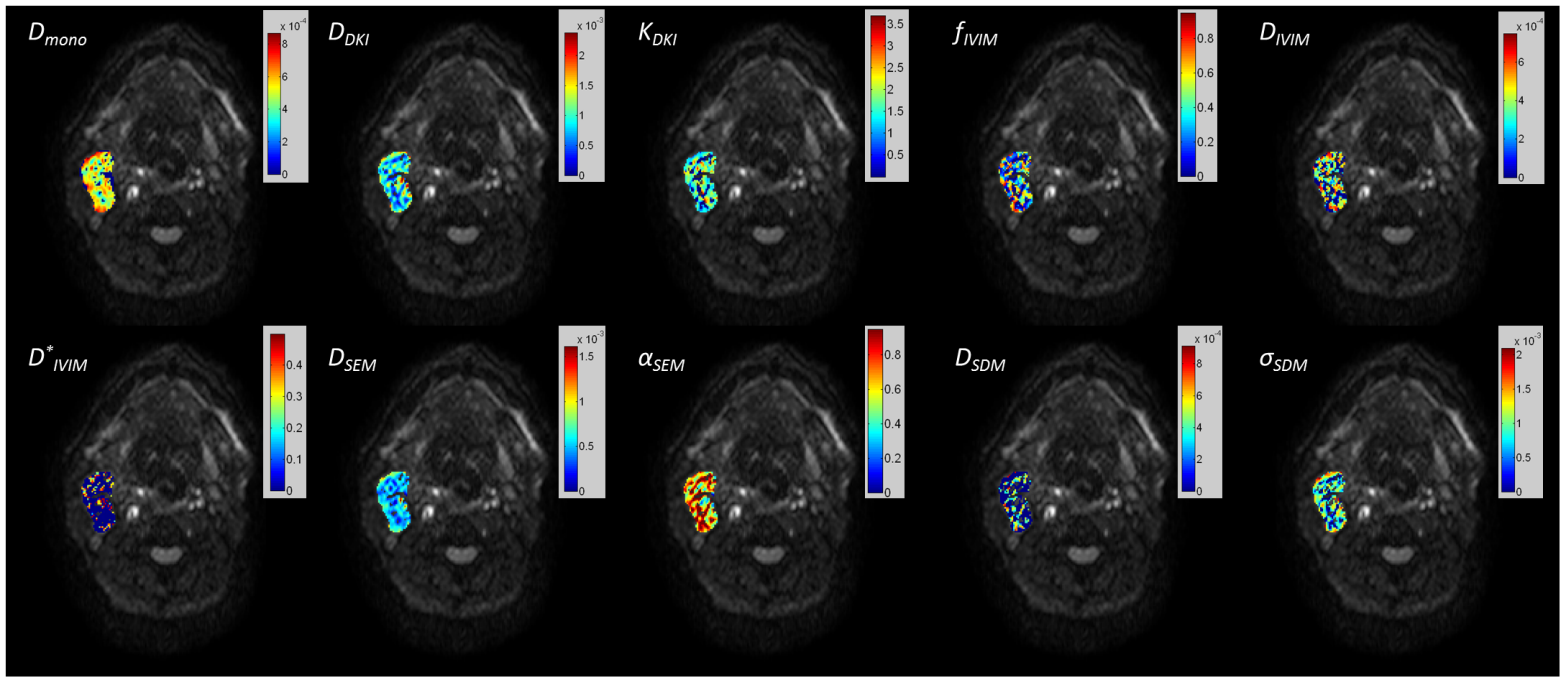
The maps of the mono-exponential apparent diffusion coefficient (ADC) and non-Gaussian parameters (goodness of fit R^2^>0.8) for the metastatic node overlaid on the DWI image with b = 0.
